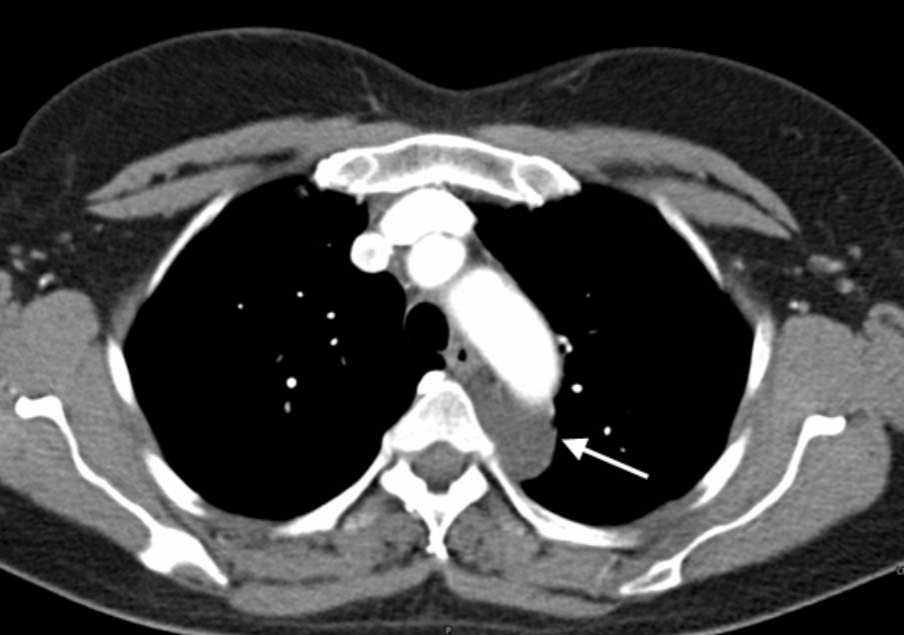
CT scan showing a cystic lesion. CT scan showing a cystic lesion (white arrow) located between the aortic arch and the thoracic spine.
